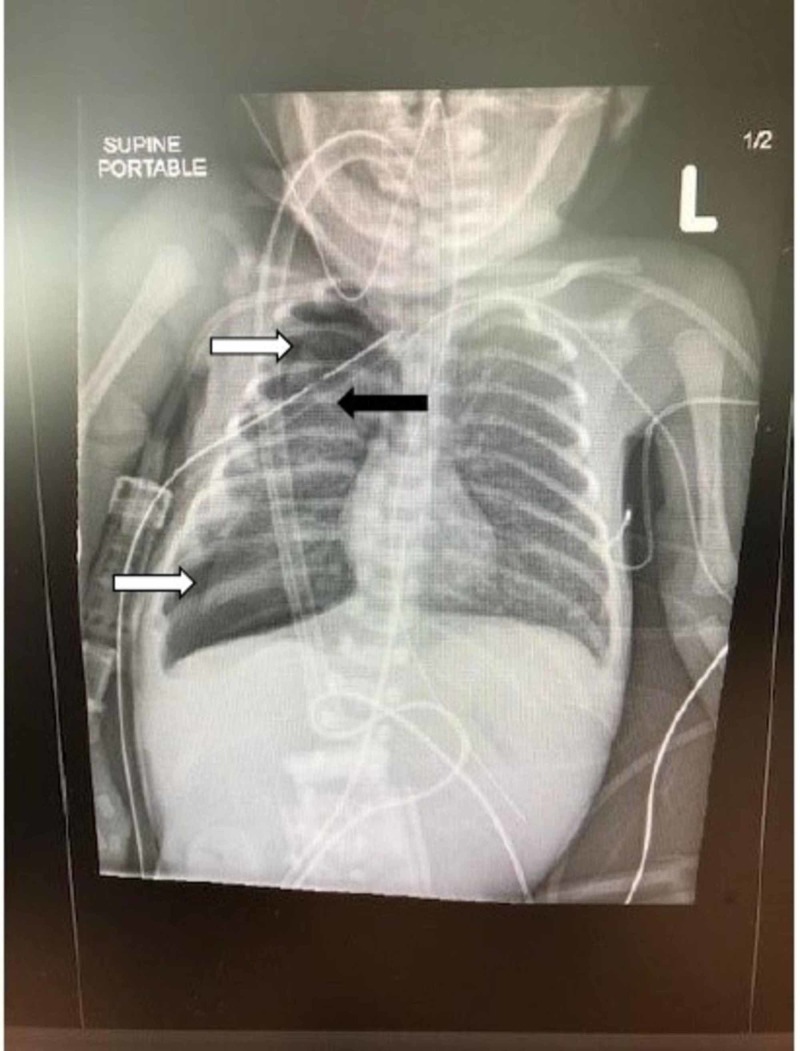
Chest X-ray obtained in the operating room Interval development of a right-sided pneumothorax (white arrows) occurred after the removal of the fragment of plastic sheath. Residual pneumothorax is demonstrated after placement of the right-sided chest tube (black arrow)
